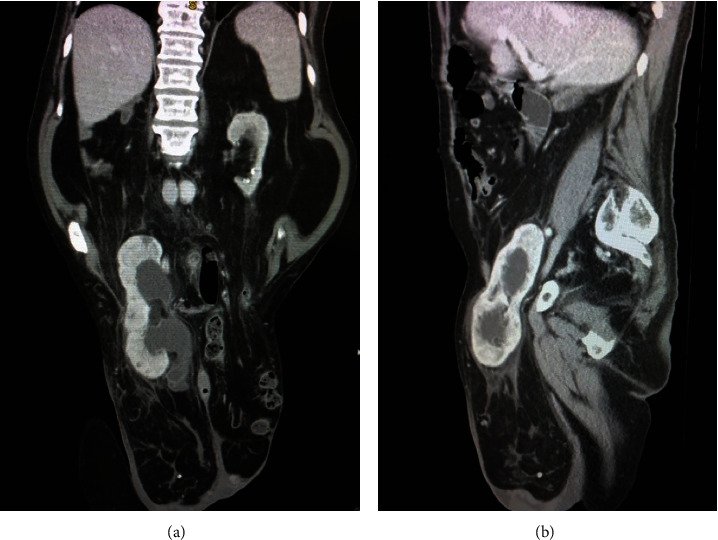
Multidetector-computed tomography (MDCT) demonstrates the location of the right kidney in the right-sided inguinal hernia. (a) Frontal reconstruction. (b) Sagittal reconstruction.
